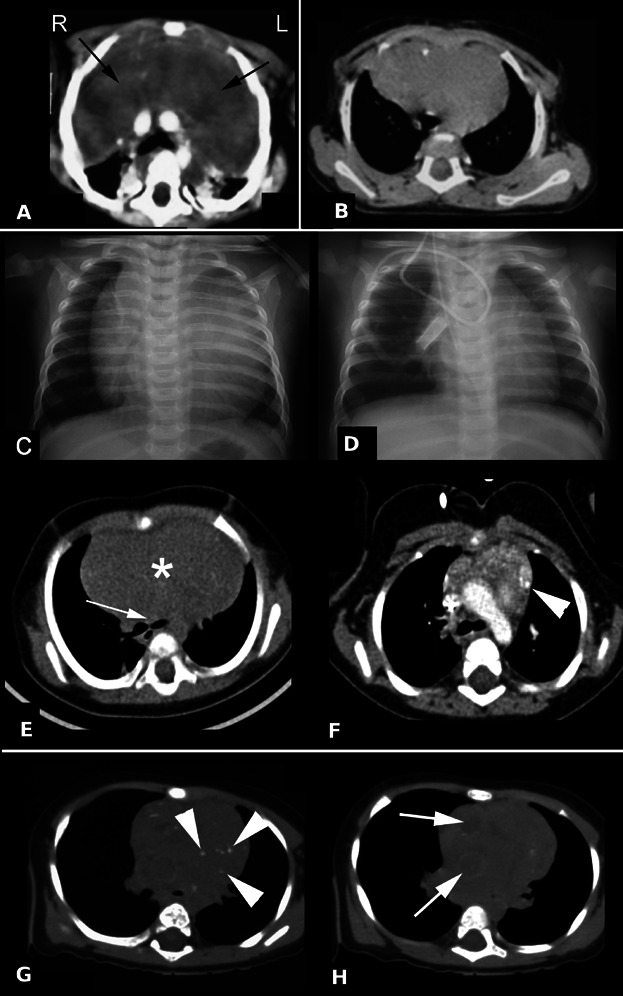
A,B: Thoracic CT of patient UPN 2106425: an 8-month-old female with respiratory distress at diagnosis. A: Huge enlargement of the thymus (black arrows). B: Evolution after a 6-week course of vinblastine and steroid; CT demonstrates decreased thymus involvement. C–F: Patient UPN 1506627, seen at 4 months of age for a skin rash. C: Chest X-rays with thymus enlargement. D: Chest X-rays after a 6-week course of vinblastine, showing normalization of thymus size. E: CT scan at time of initial diagnosis with thymus enlargement (*) and tracheal pushback (white arrow). F: Evolution after a 6-week course of vinblastine and steroid, demonstrating the decreased thymus size, but calcifications are now present (arrowhead). G,H: Thoracic CT of patient UPN 1506102. G: Presence of multiple calcifications (arrowheads). H: Coexistence of intra-thymic cysts (white arrows).
